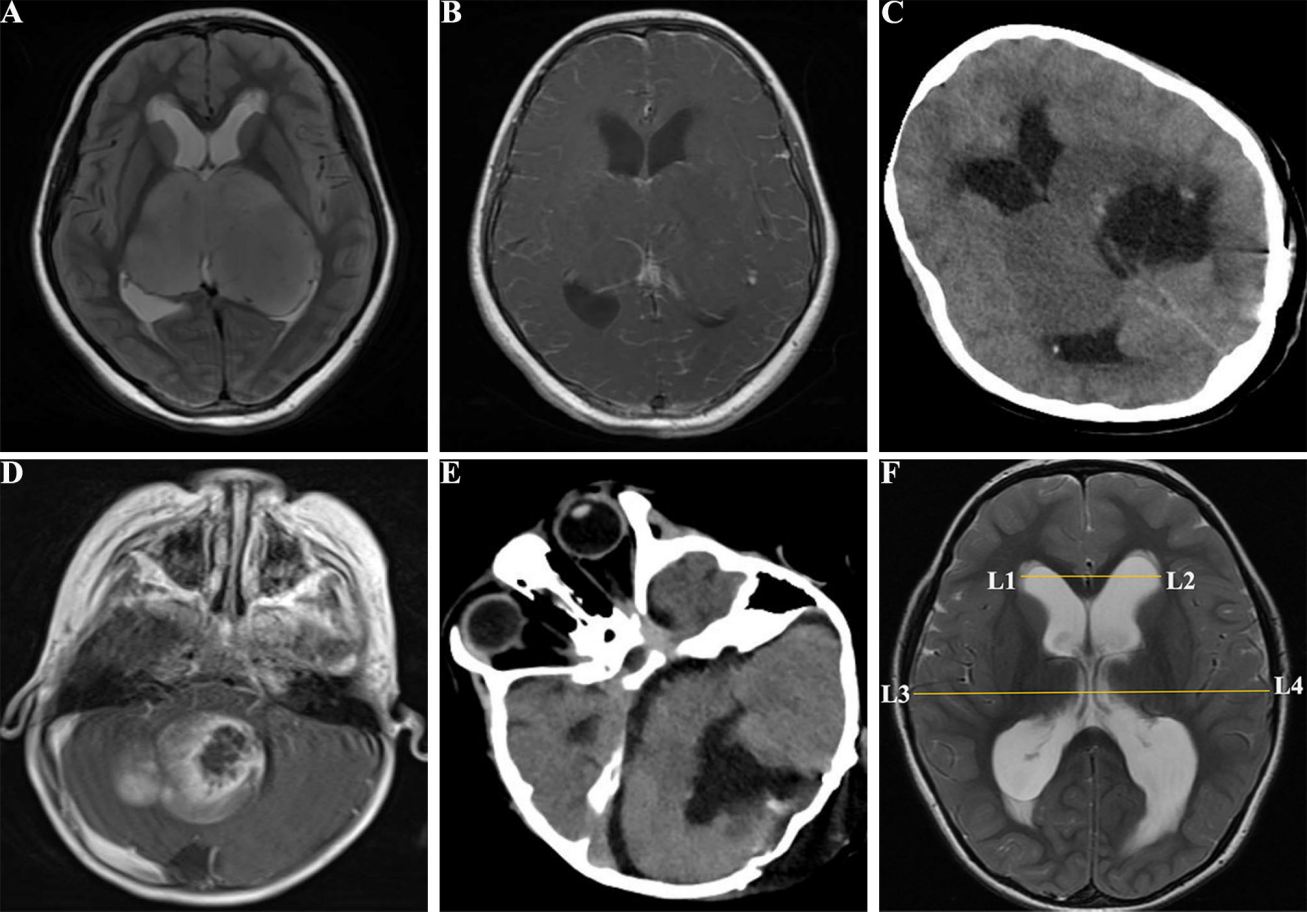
Images of pediatric patients with brain tumors. (A), a 10 years old female with supratentorial tumors in the T2 FSE of MRI before surgery; (B), enhanced MRI T1 FSE+C showed bilateral supratentorial tumors before surgery; (C), CT image of the supratentorial tumor with incomplete total resection; (D), a 3 years old male with infratentorial tumors in the enhanced MRI T1 FSE+C before surgery; (E), CT image of the infratentorial tumor after tumor resection; (F), Postoperative hydrocephalus with Evan’s index > 0.3. FSE, fast spin echo; CT, compute tomography; MRI, magnetic resonance imaging; Evan’s index = L1L2/L3L4.
